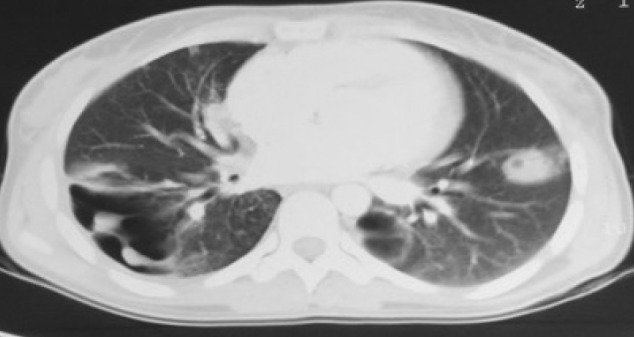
Computed tomography chest scan showing multiple thick-walled
